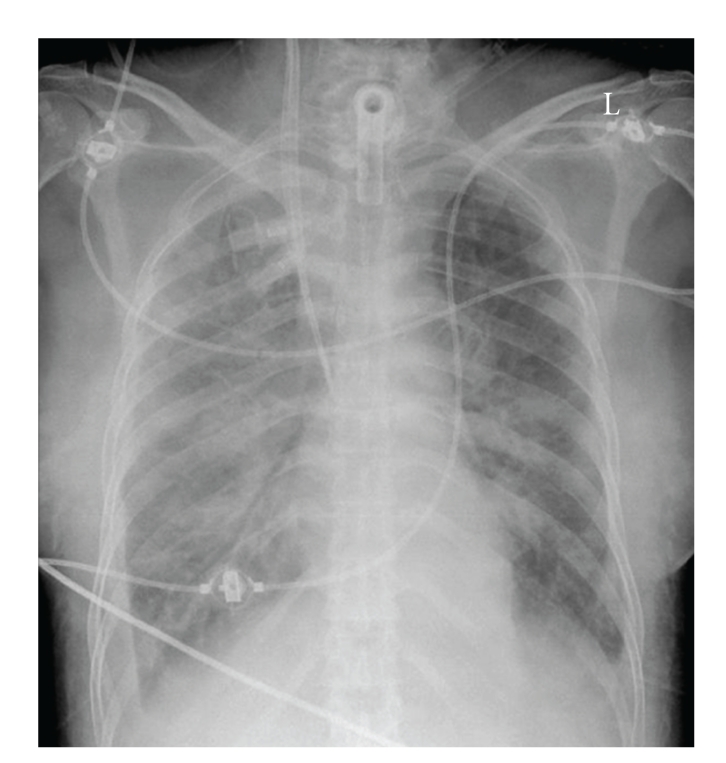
The chest X-ray on ICU admission reveals pulmonary edema.
